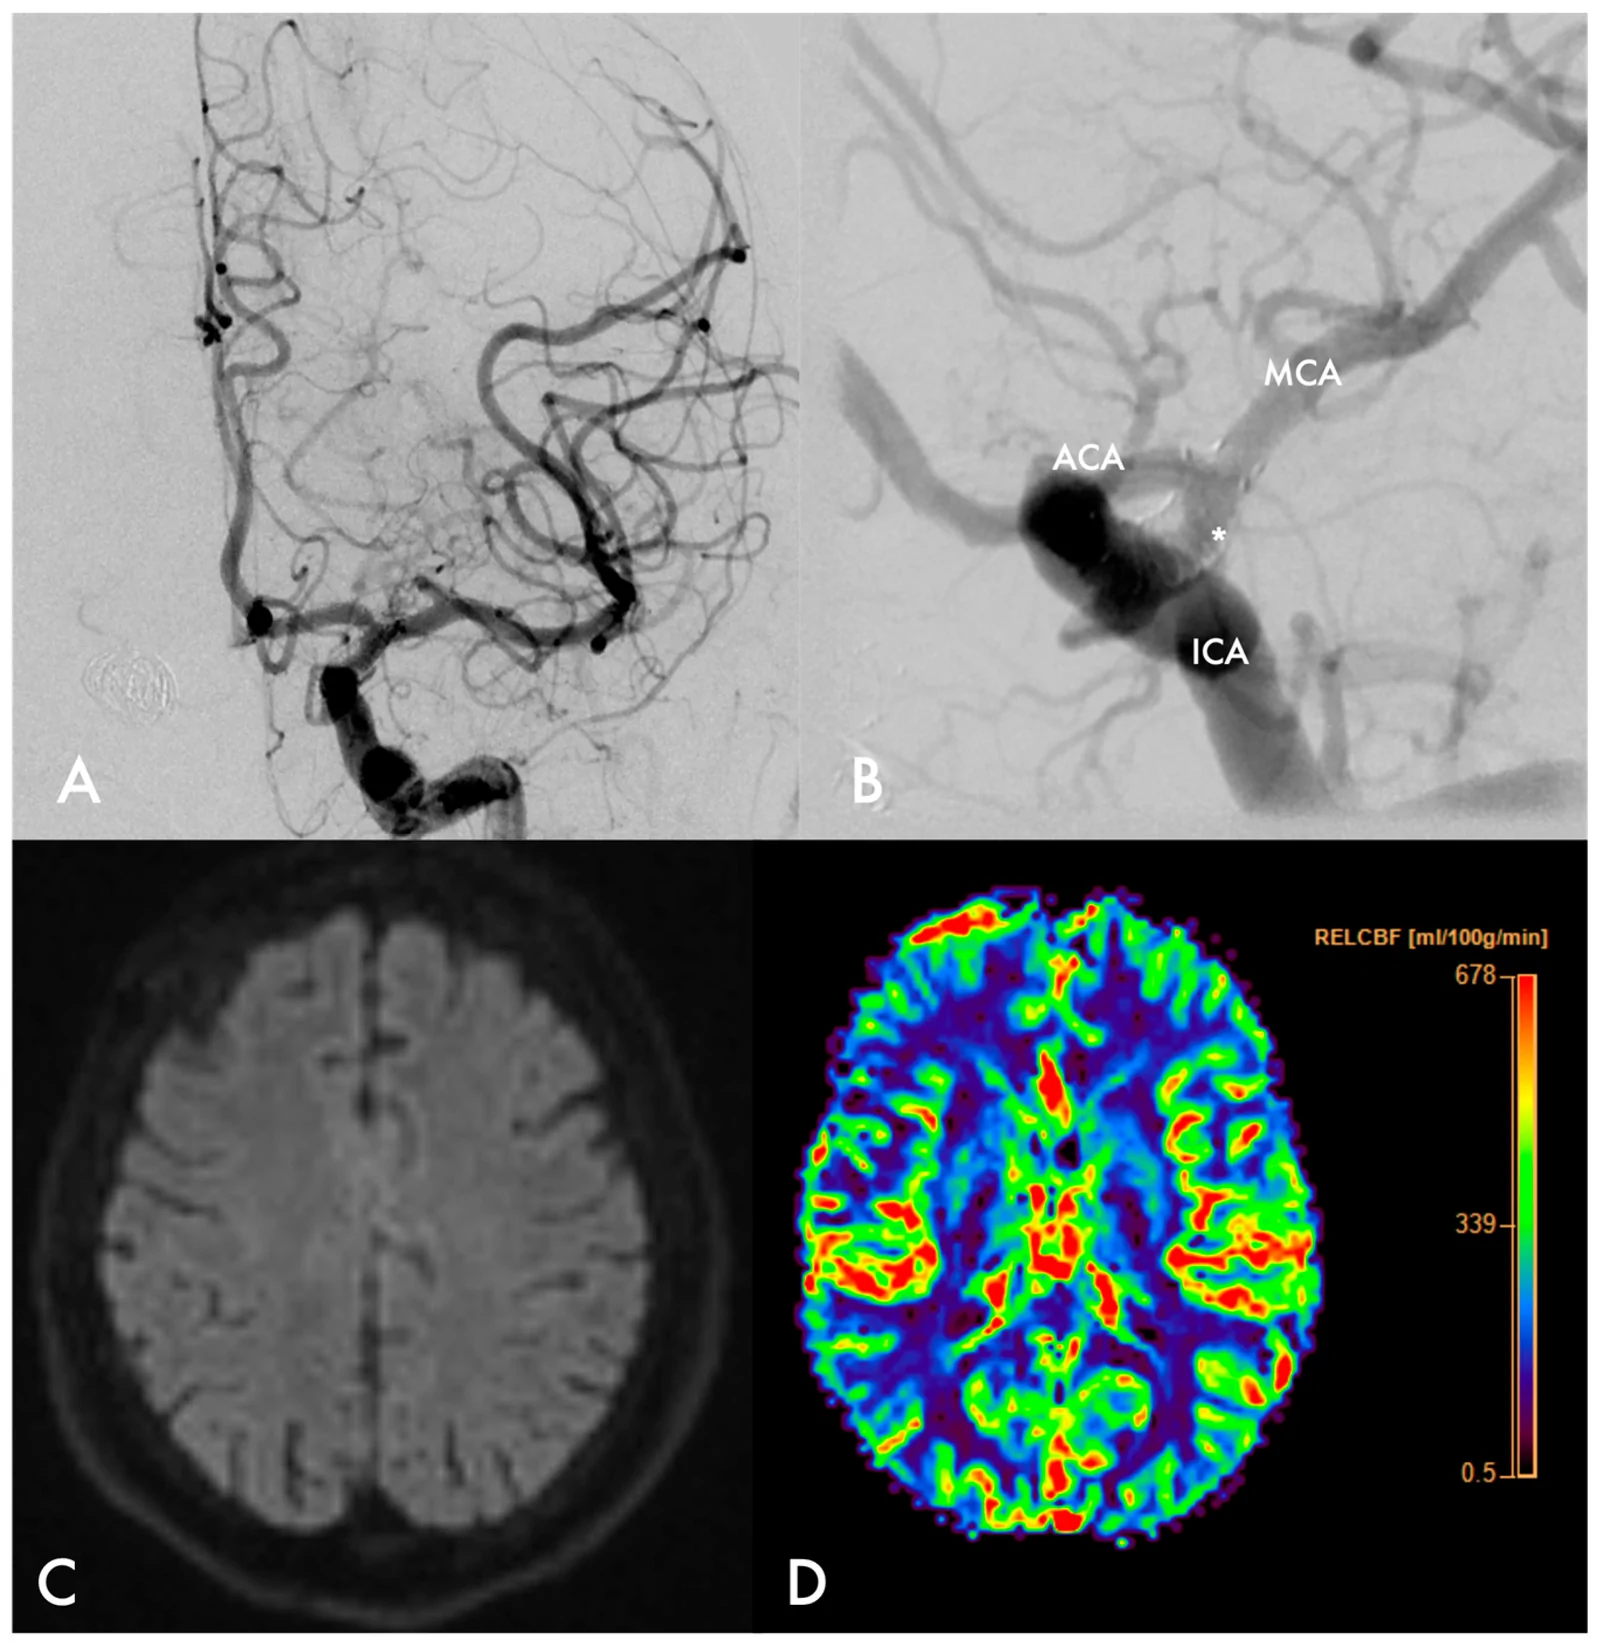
After administration of tirofiban, sufficient perfusion is achieved throughout the ICA, as well as in the ACA and MCA. The stent (asterisk) appears unchanged in position and patent (A,B). Follow-up MRI on postoperative day 2 shows no areas of restriction on diffusion-weighted sequences (C). The CTP study demonstrates normal CBF in the left hemisphere (D).
